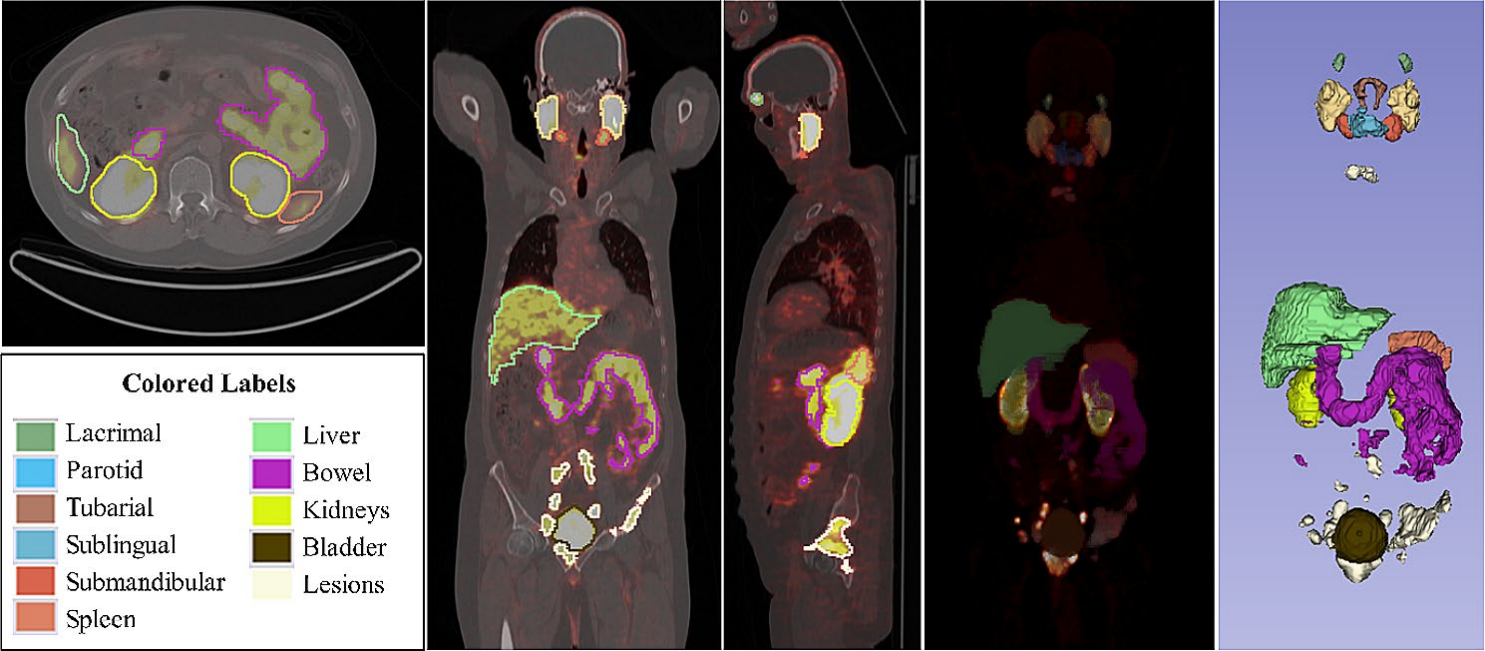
[^68^Ga]Ga-PSMA-11 PET/CT image of a mCRPC patient with segmented targets in transaxial, coronal, sagittal, MIP, and 3D views from left to right using 3DSlicer 5.2.2 software
